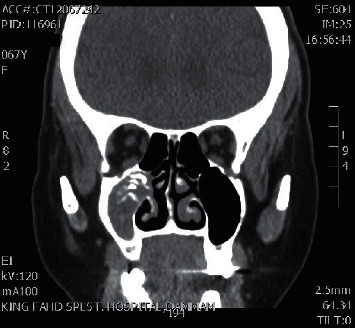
Coronal CT sinuses showing right maxillary sinus fungal ball.
